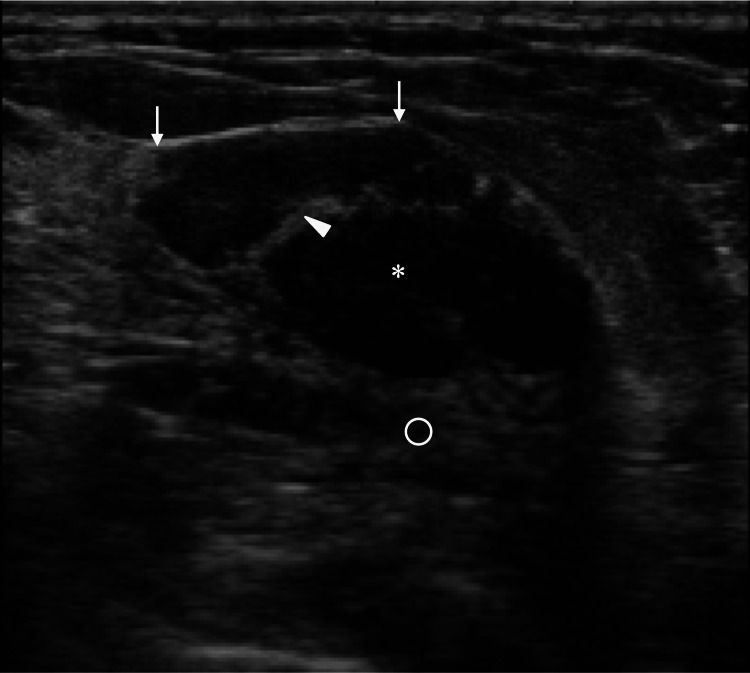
Ultrasound findings Ultrasound showed a well-circumscribed mass (asterisk) with predominantly low internal echoes, internal high echoes in very limited areas (arrowhead), and enhanced posterior echoes (open circle). Interfaces between the mass and the surrounding fat showed very strong high echoes at the mass margins parallel to the overlying skin (arrows).
